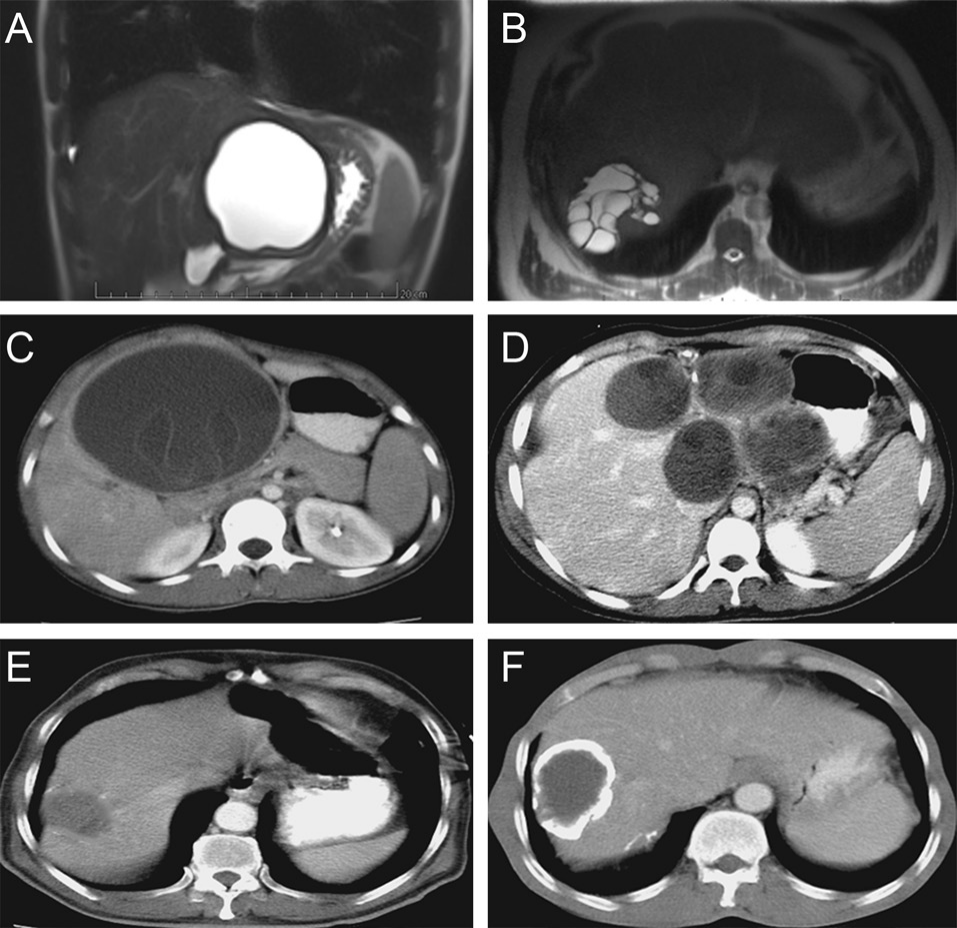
Demonstration of differential diagnosis of cystic echinococcosis by computed tomography (CT) and magnetic resonance imaging (MRI) according to the cyst stage. (A) Hyperintense hepatic cyst with double-line sign considered pathognomonic for World Health Organization (WHO) CE1 cysts, T2-weighted MRI. (B) Multivesicular cystic echinococcosis hepatic cyst WHO CE2 cyst, T2-weighted MRI. (C) Hypodens hepatic cyst with detached endocyst (water-lily sign) WHO CE3A cyst, CT. (D) Daughter cysts inside a solid cyst matrix WHO CE3B cyst, CT. (E) Heterogeneous, parasitic membranes embedded in avascular solid contents WHO CE4 cyst, CT. (F) Cysts with degenerative content and heavily calcified wall WHO CE5 cyst, CT.
